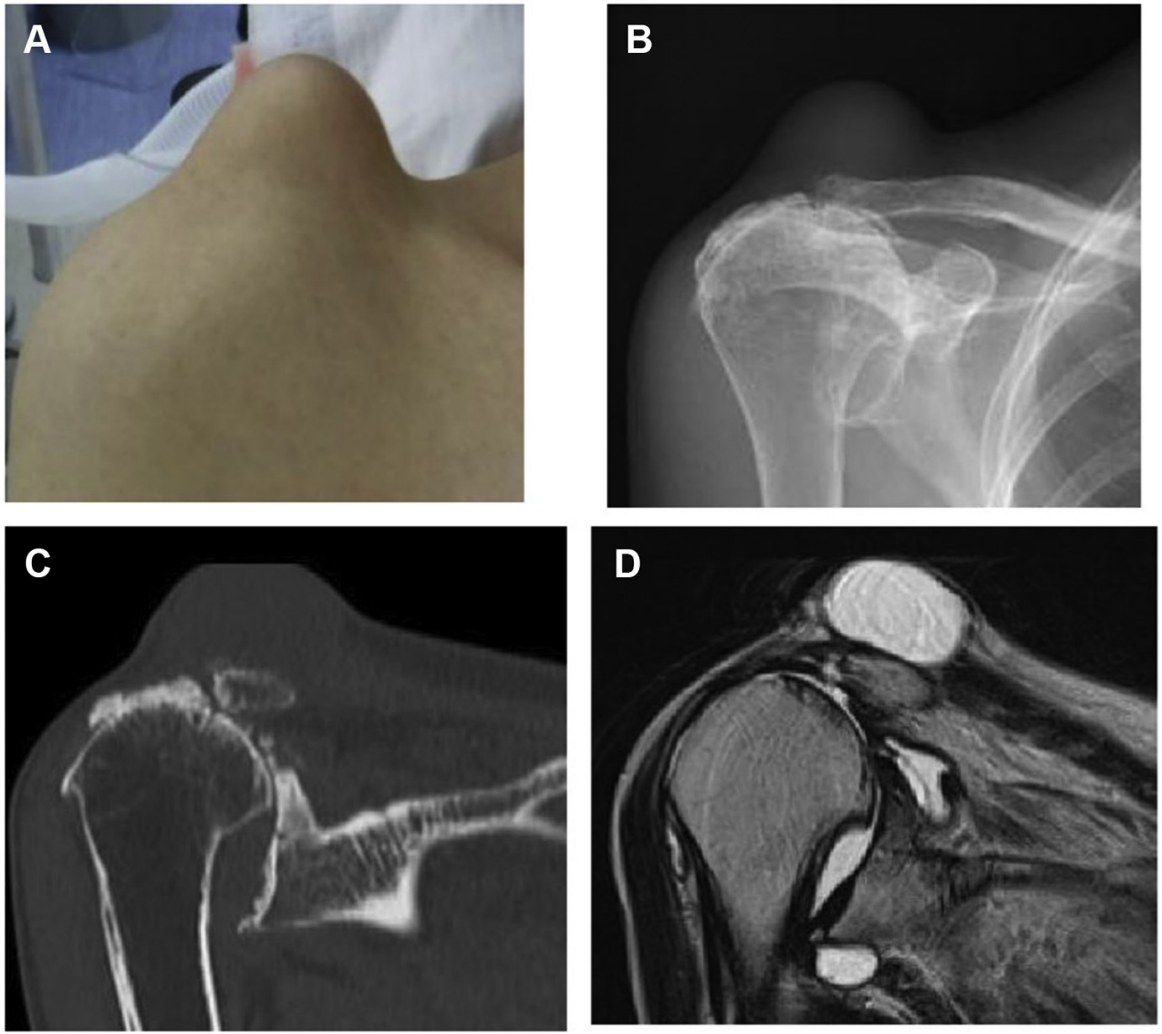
Preoperative swelling of the acromioclavicular joint and preoperative images of Case 2. (A) There is notable swelling of the acromioclavicular joint. (B, C) The humeral head is translated superiorly, and the acromioclavicular joint shows degeneration. (D) MRI scan shows a cyst in the superior part of the acromioclavicular joint. *MRI*, magnetic resonance imaging.
